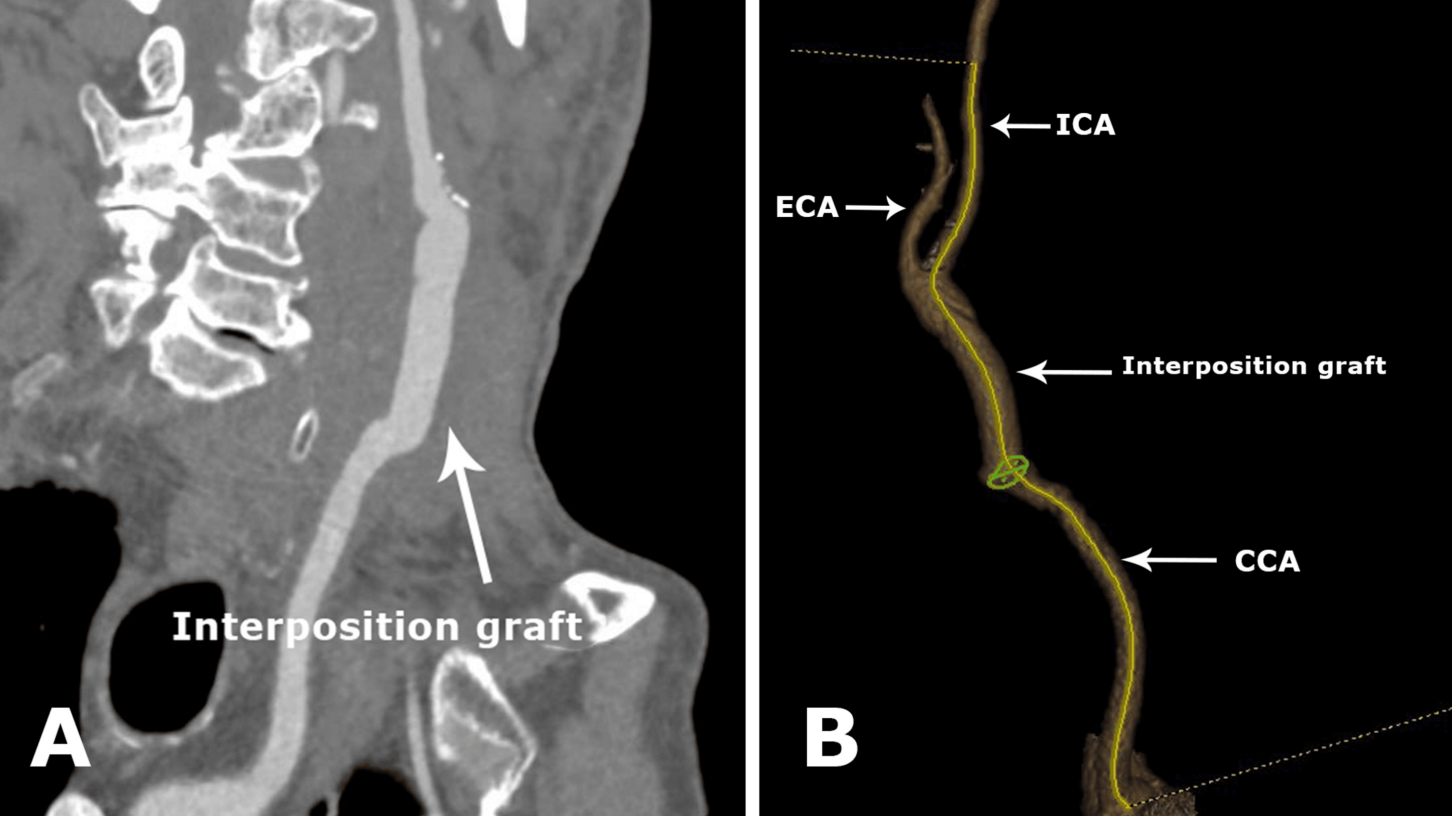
Postoperative computed tomography angiogram (CTA) showing patent reconstruction. A) 2D reconstruction of the interposition graft.
